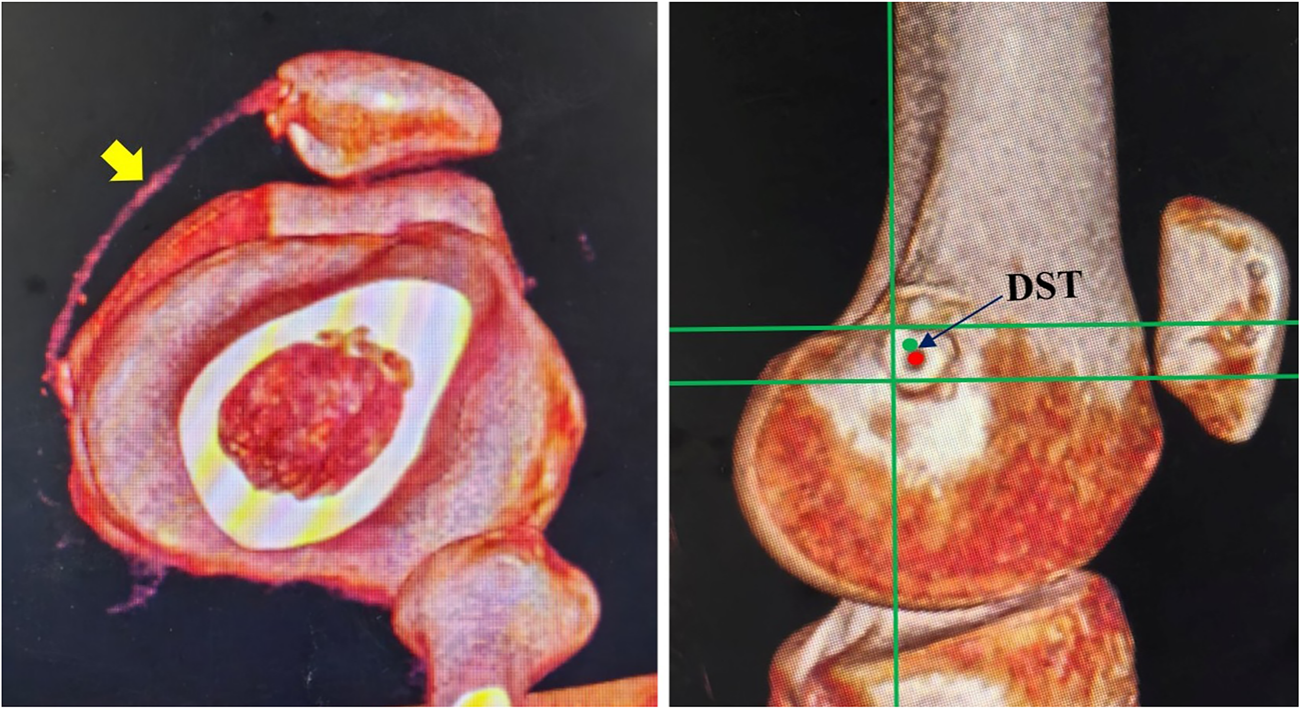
Post‐operative CT reconstruction revealed patella reduction, a reconstructed MPFL (yellow arrow) and DST measurement (The green dot indicates the Schöttle point, while the red dot indicates the actual femoral insertion.). CT, computed tomography; DST, the distance between the Schöttle point and femoral tunnel insertion; MPFL, medial patellofemoral ligament.
